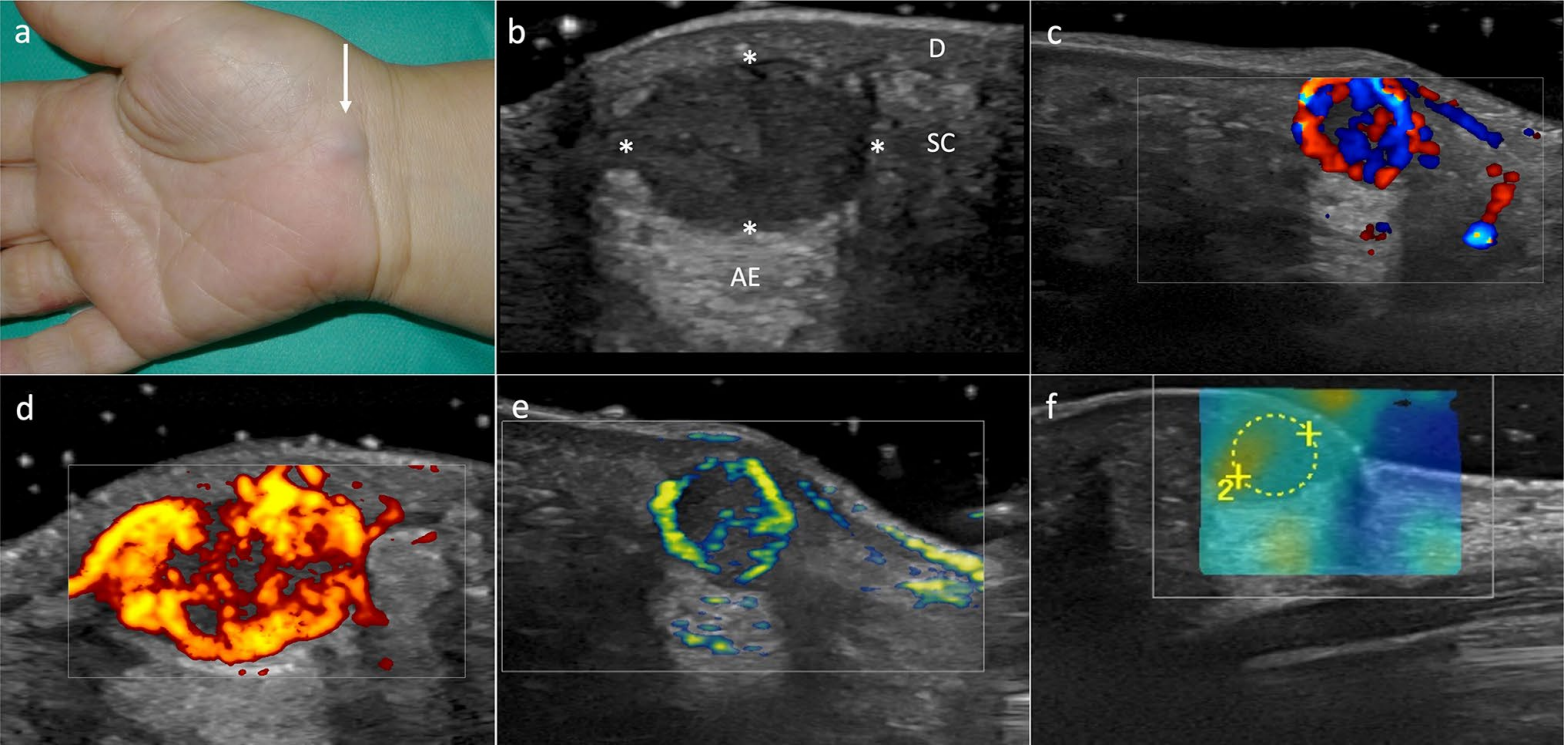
a Subcutaneous nodular lesion with a bluish discoloration located on the anterior region of the right wrist (arrow). b–d Sonographic study of the lesion. b A well-defined, non-encapsulated, hypoechoic, round dermal (D)-subcutaneous (SC) lesion (*) with posterior acoustic enhancement (AE). c Color Doppler, d power Doppler and e elastography studies
